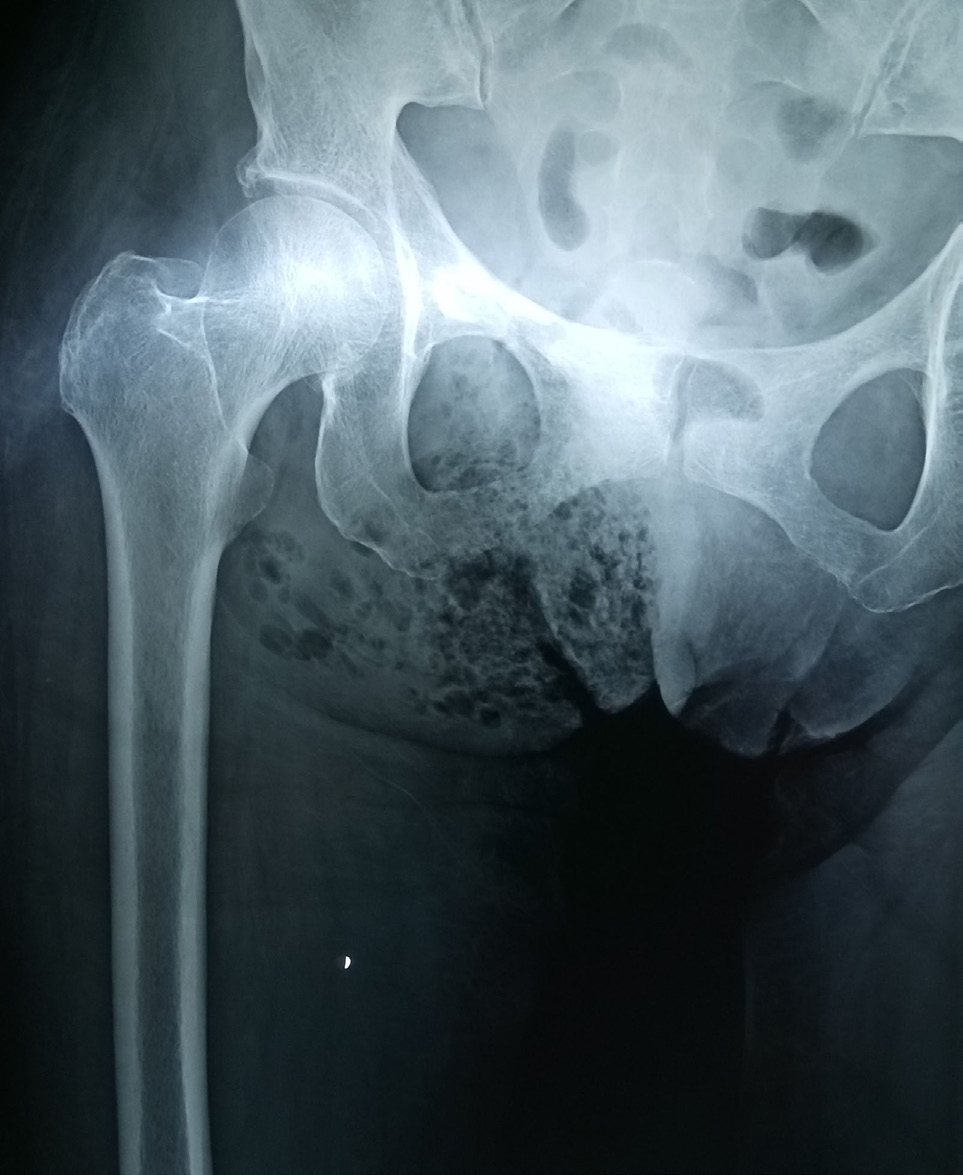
Pelvic X-ray showing the presence of air in the soft tissue.
